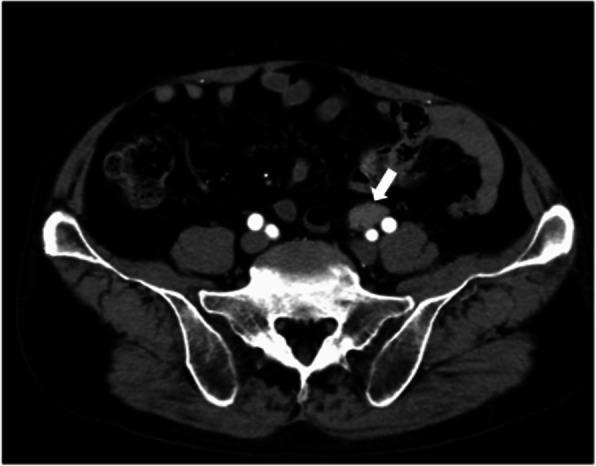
Enhanced abdominal computed tomography (CT) findings. Enhanced abdominal CT showed a 30-mm soft-tissue mass involving the left ureter in front of the bifurcation of the left common iliac artery (arrow)
